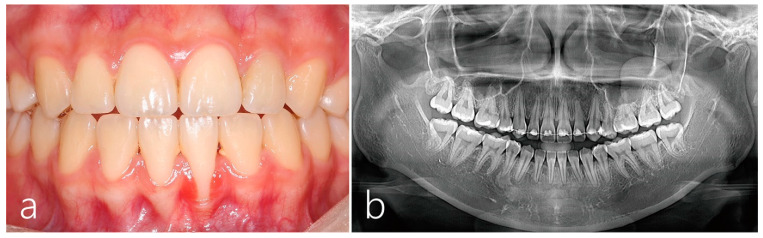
(a) Clinical finding 2 years after orthodontic treatment showed gingival recession in the left lower central incisor with a thin periodontal biotype. The roots of lower incisors were slightly labially protruded, and the interdental areas were relatively depressed. (b) Root resorption and interdental bone loss of lower incisors were observed on panoramic radiography at 2 years after orthodontic treatment.
